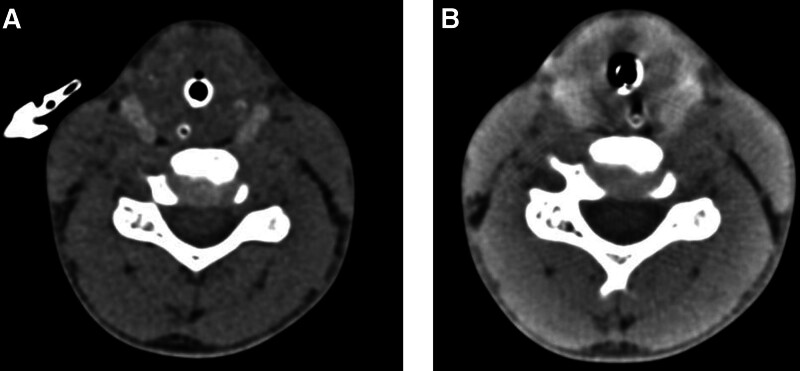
(A) A CT scan of the neck revealed significant bilateral soft tissue swelling in the subglottic region. (B) Follow-up CT imaging of the neck indicated a notable decrease in subglottic soft tissue swelling compared to the previous scan. CT = computed tomography.
